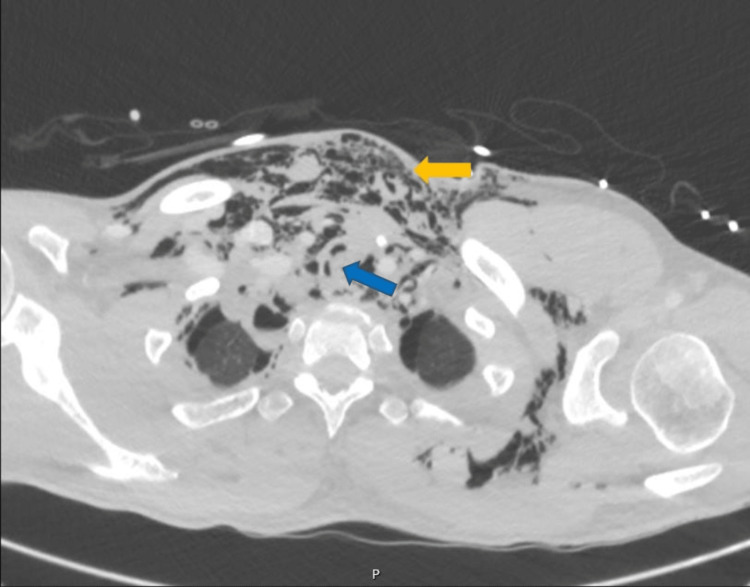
First ICU day thoracic CT: Marked reduction of the tracheal lumen, less than 3 mm (blue arrow), more expressive subcutaneous emphysema (yellow arrow)
